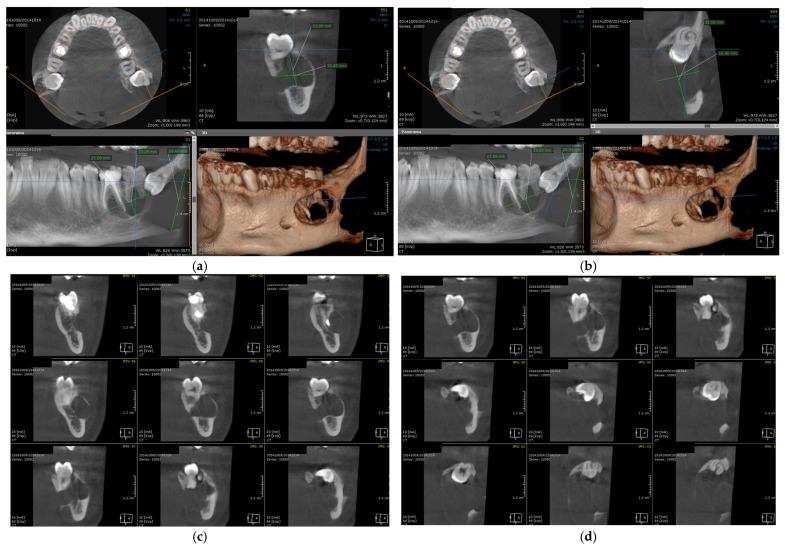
Initial CBCT, different aspects showing the extent of the tumor, in axial, sagittal, frontal and reconstruction view (a,b) and 2 mm slices in axial view (c,d).
